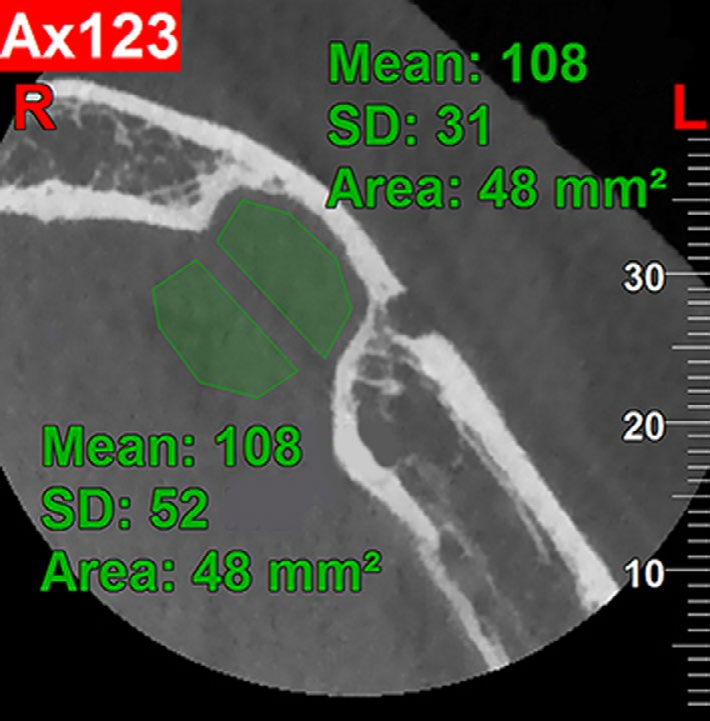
Axial CBCT section: The adjacent lingual soft tissue presented the mean density (gray scale value) similar to that of the lingual depression
